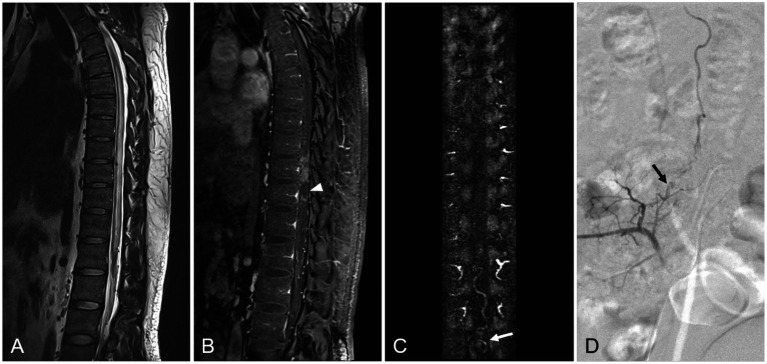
A 70-year-old patient with sacral SDAVF. (A) Sagittal T2-weighted image reveals a signal intensity increase of spinal cord, involving the conus. (B) The enhanced T1-weighted image demonstrates the patch unenhanced region in the spinal cord (*white arrowhead*). (C) In the coronal MRA MIP image, the dilated filum terminale vein suggests that the feeding artery is probably located in the sacrum (*white arrow*). (D) DSA examinations confirm that the fistula supplied via the right internal iliac artery (*black arrow*).
